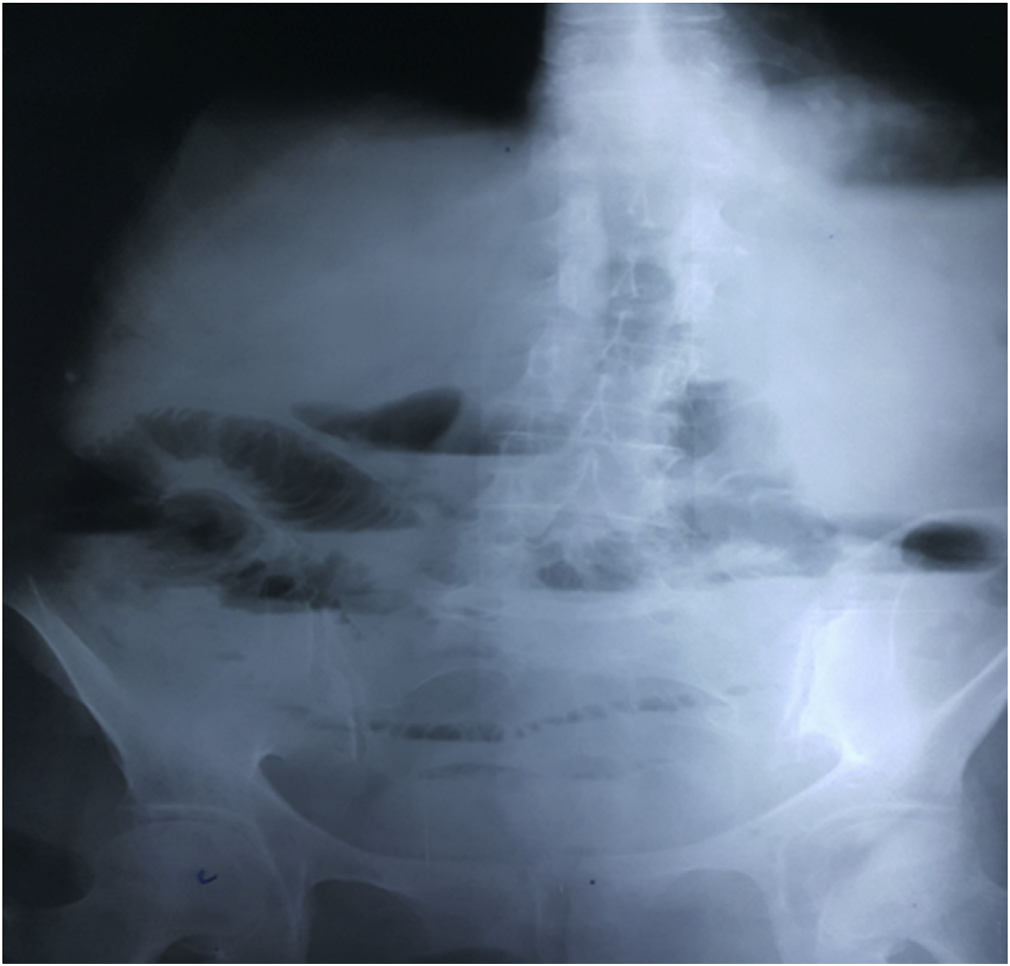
Plain abdominal X-ray showing increased air-fluid levels without air in the rectum.
